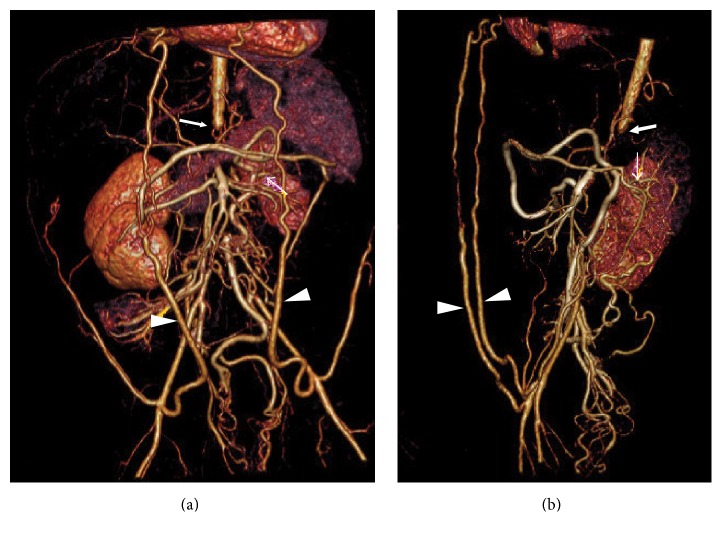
CT angiogram (3D reconstruction): (a) anterior; (b) lateral. The abdominal aorta is occluded at T12 (thick arrow). Both internal mammary arteries are markedly enlarged and ramify with the inferior epigastric arteries (arrowheads). The renal arteries arise from collaterals inferior to the termination of the aorta. The left renal artery (thin arrow) is small and there is decreased enhancement of the anterior half of the left kidney.
